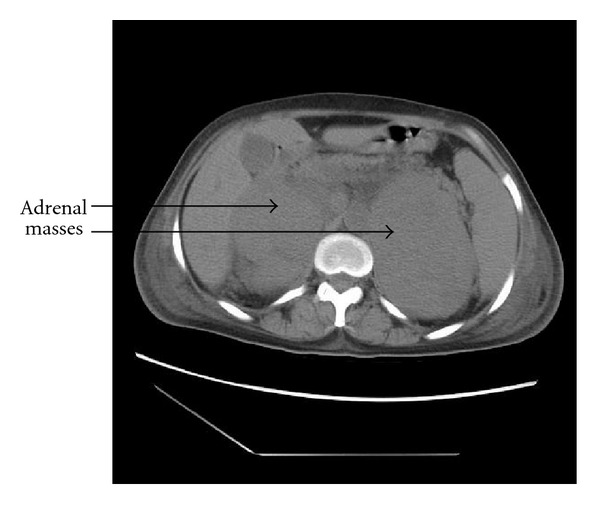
The abdominal CT scan showed an increase of the volume of adrenal masses.
